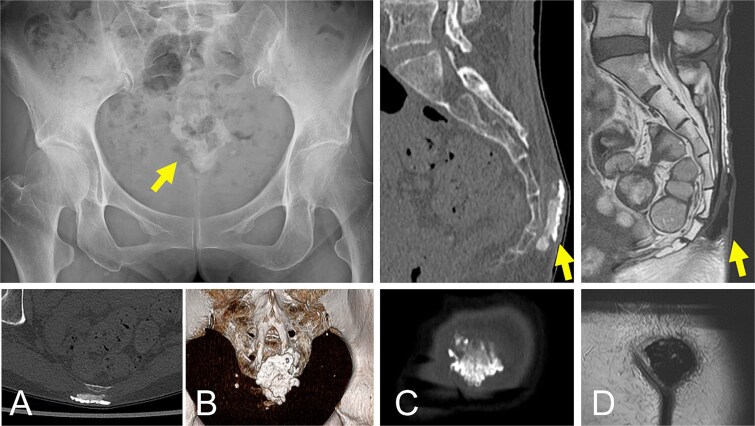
Plain radiographs (A) and CT (A-bottom, B and C) show a calcified mass in the sacral region (arrows). MRI demonstrates a lesion with low-to-high signal intensity on T1-weighted images (D) (arrows).
